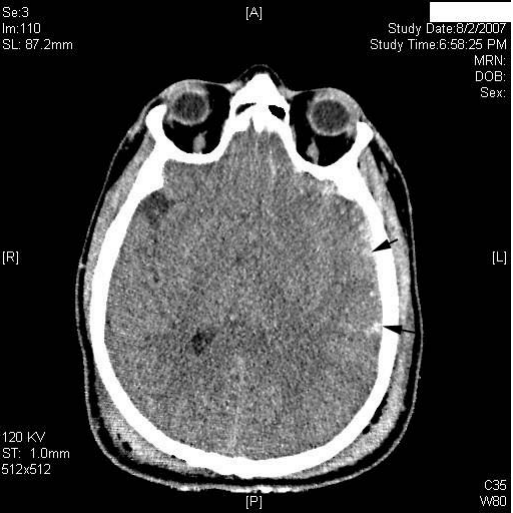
Computed tomography scan at the level of the orbits. Punctiform contusions involving the left temporal and frontal lobes with effacement of the left occipital horn are demonstrated (arrows). A = anterior; P = posterior; L = left; R = right.
